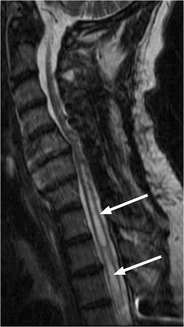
Syrinx: T2-weighted sagittal image from cervical spine MRI that shows expansion of the spinal cord with central cyst formation extending from the C6 level into the upper thoracic spine (*white arrows*)
